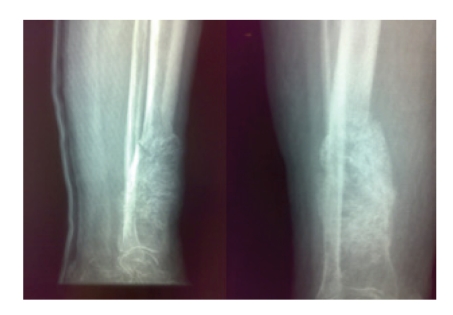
Radiograph of the tibial metastasis showing the increased dimensions and the soft-tissue mass.
